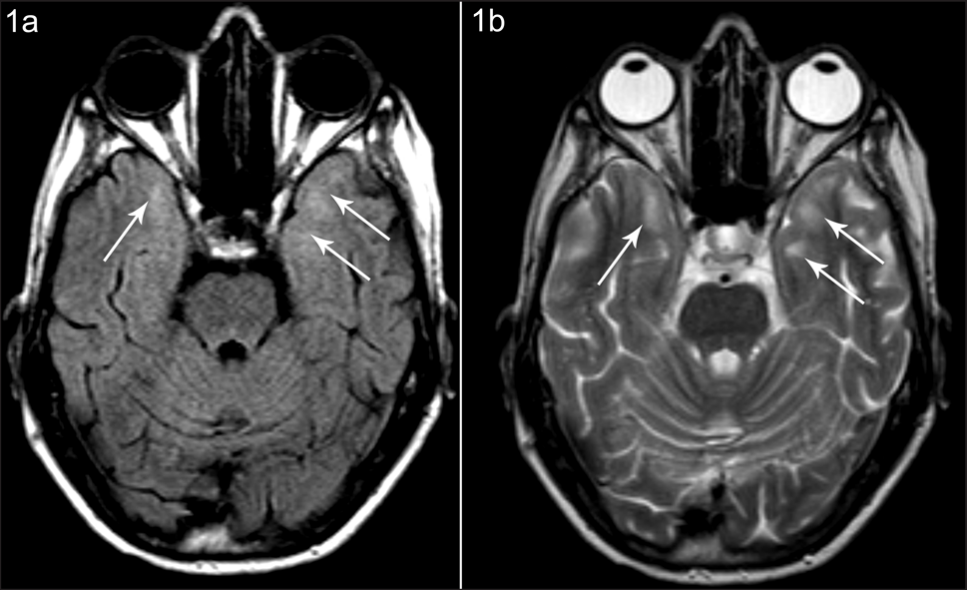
Patient A, axial FLAIR (a) and T2-weighted (b) images at the same level show subcortical white matter hyperintensity anteromedial in the temporal lobes, more pronounced on the left side.
